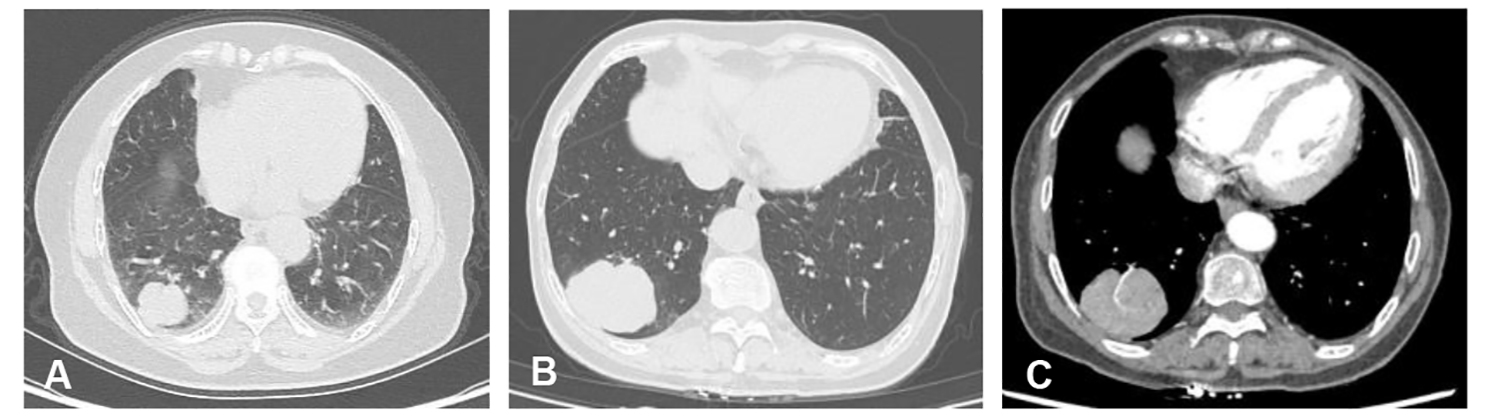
The chest CT scan manifestation of the tumor. A, In 2018, the tumor was well circumscribed, lobulated, and the maximum diameter was 3.7 cm. B, In 2021, the maximum diameter of the mass was 5.5 cm, with more obvious lobulation. C, Blood vessel was visible in the arterial phase of the tumor
